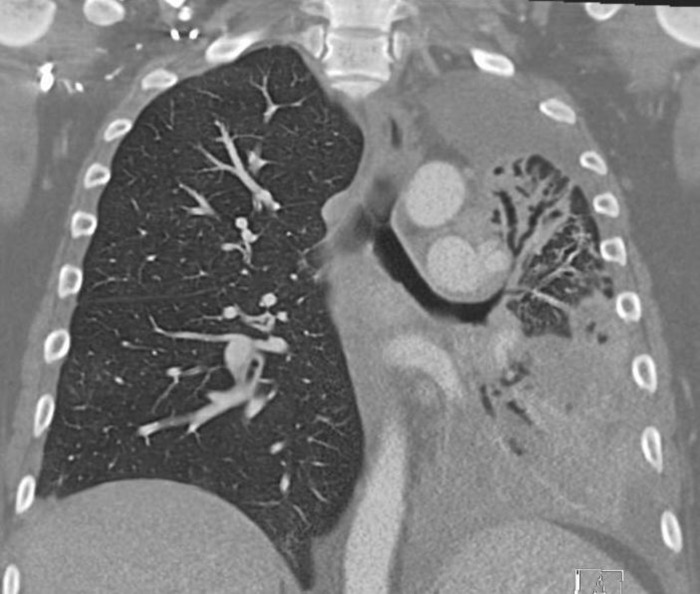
Coronal section on CT chest showing endobronchial lesion with extrinsic compression of the left main stem bronchus with post-obstructive atlectasis
